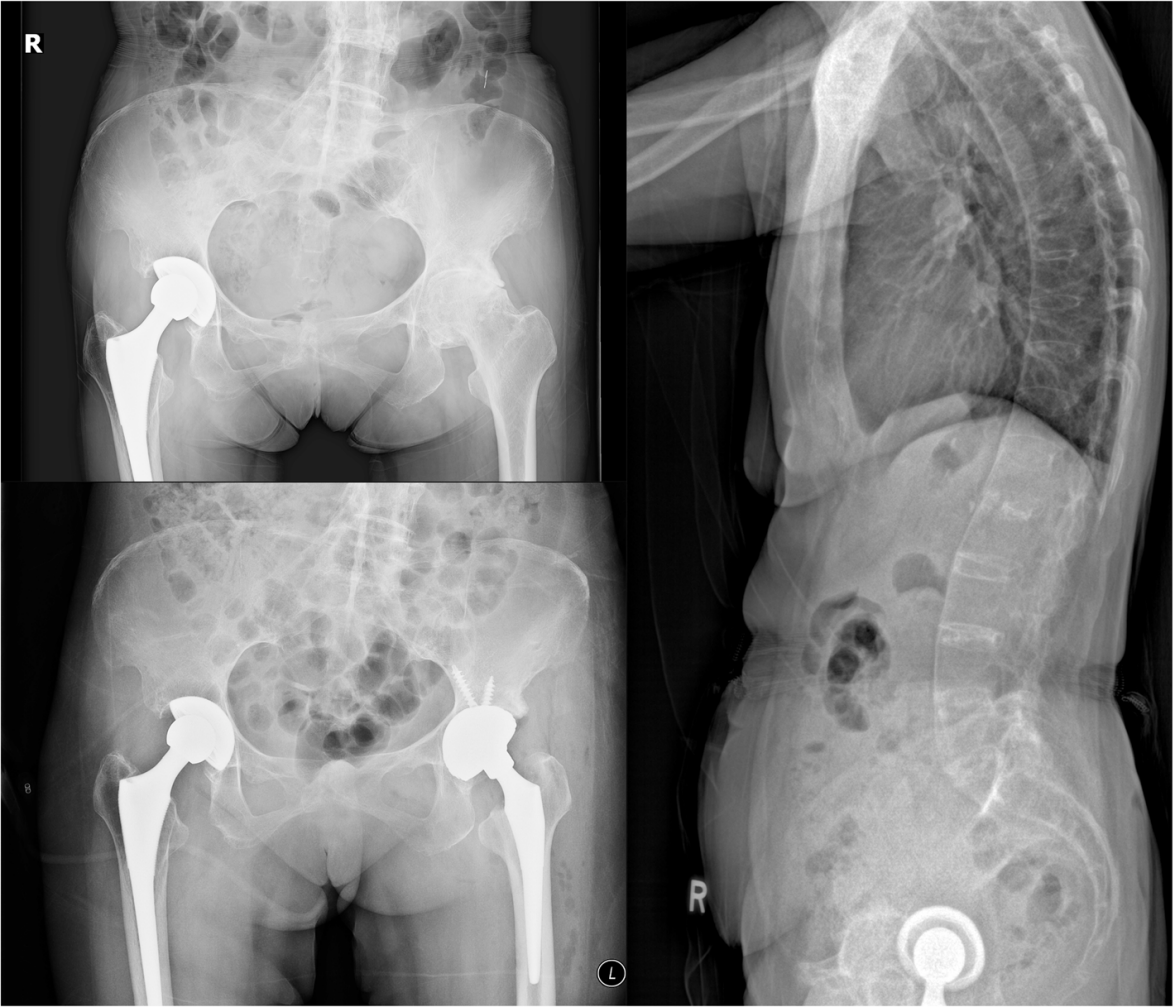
Case No. 6 in Table 1. a Lateral X-ray showing whole spine spontaneous fusion because of inflammatory arthritis. b Osteoarthritis on the left hip. c DM THA on the left hip with spinopelvic imbalance
